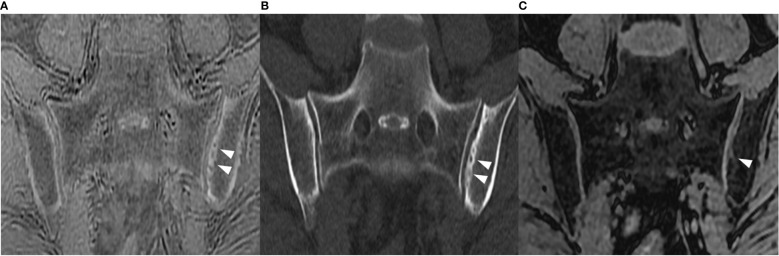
Semi-coronal images in a 30-year-old woman with sacroiliitis. (A) ZTE, (B) ldCT, and (C) LAVA-Flex. Obvious second-degree erosions on the left iliac surface could be clearly exhibited on ZTE and ldCT (white arrowhead) but only graded as first-degree on LAVA-Flex. ldCT, low-dose CT; ZTE, Zero echo time; LAVA-Flex, Liver Acquisition with Volume Acceleration-flexible.
